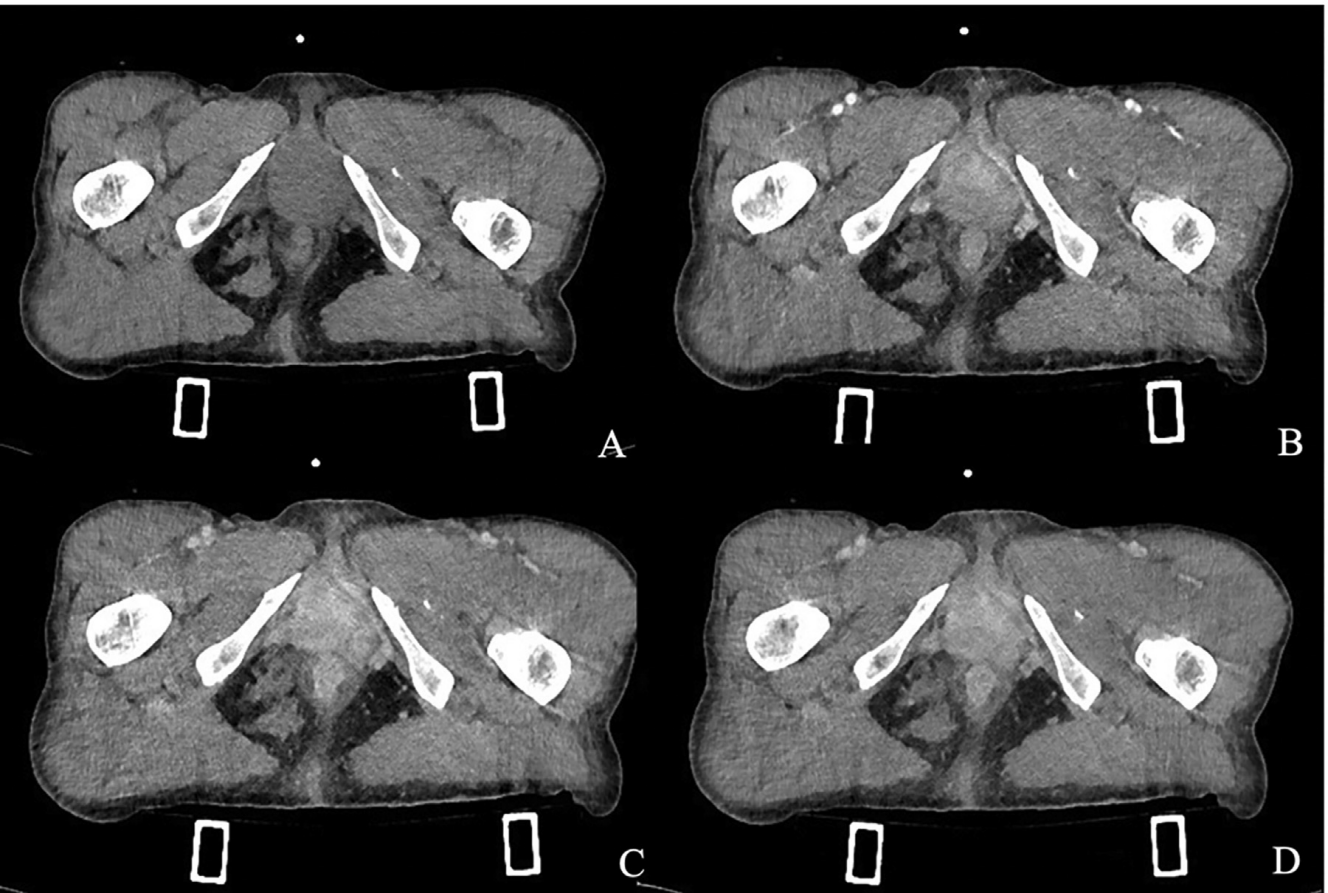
(A–C) CT axial scan showing phase without contrast (A), during the arterial phase (B), the nephrographic phase (C) and delayed phase (D) demonstrating suprafluid-blood distention of the vagina and thickening and imbibition of the adjacent soft tissues.
